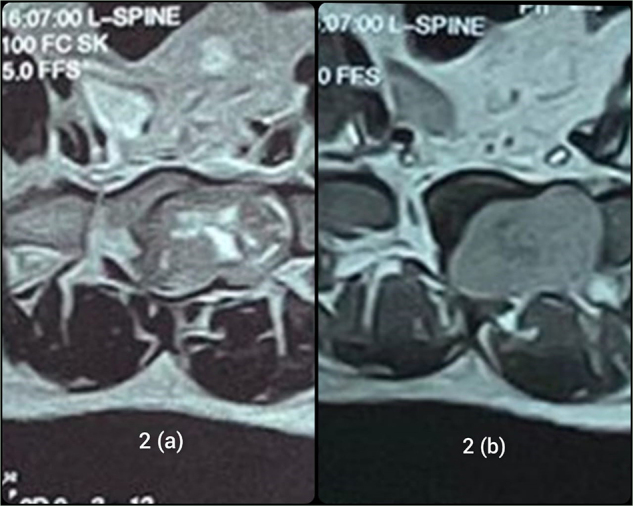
(a) and (b) show the axial views of T2- image and T1-weighted image, respectively.
